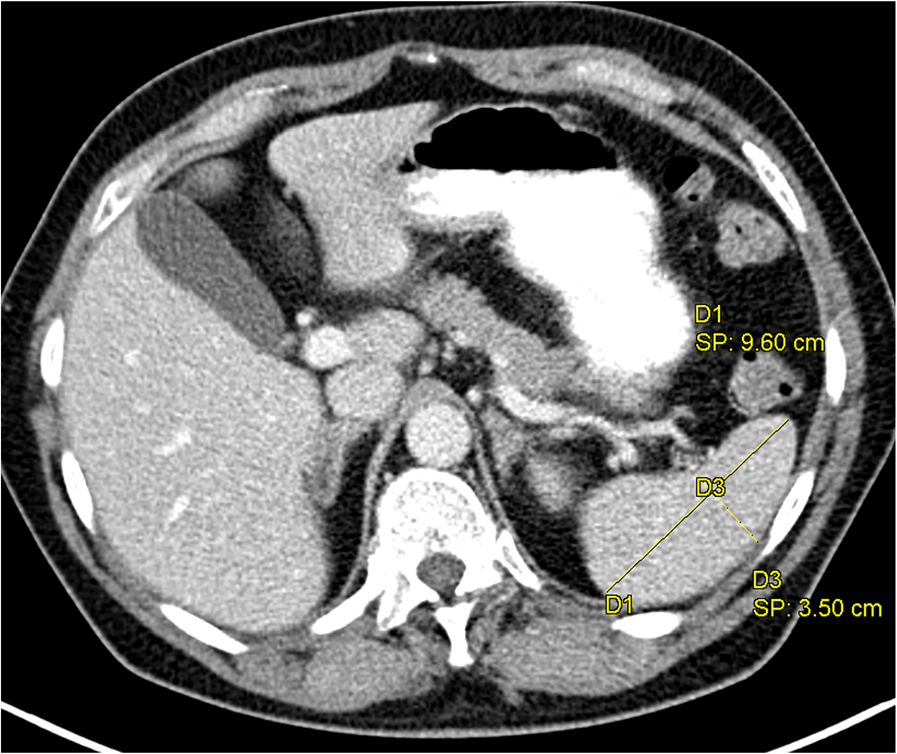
Example of a normal spleen. An axial image of a contrast-enhanced computed tomography examination of the upper abdomen. The size of the spleen in the axial plane is specified by measuring the length and width at the hilum
